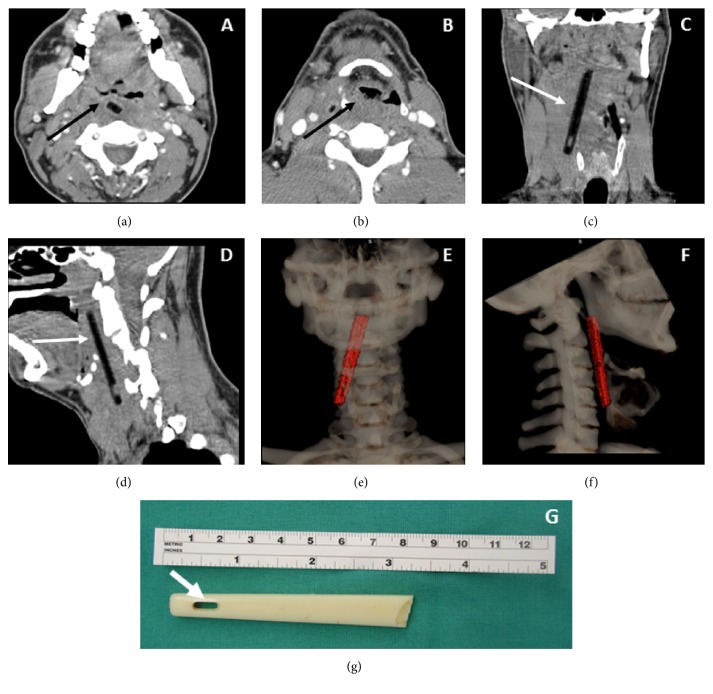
Two-dimensional axial (a, b), coronal (c), and sagittal (d) CT images and 3-dimensional coronal (e) and sagittal (f) reformats show a low density linear structure on the 2-dimensional images (white arrow) which is outlined in red on the 3-dimensional images. It is imbedded within the retropharyngeal soft tissues and extends from the level of the oropharynx through the hypopharynx to the right lateral extrapharyngeal soft tissues (black arrow). There is a linear gap at the end of the foreign body which represents the opening within the toothbrush handle (arrow (g)).
